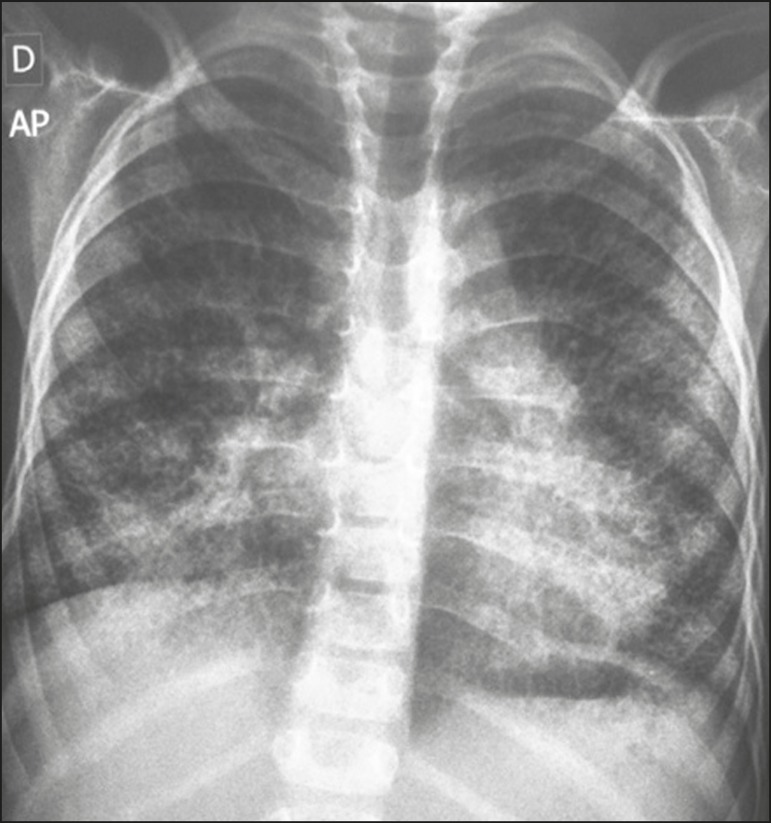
Anteroposterior chest X-ray showing a bilateral reticulonodular interstitial
